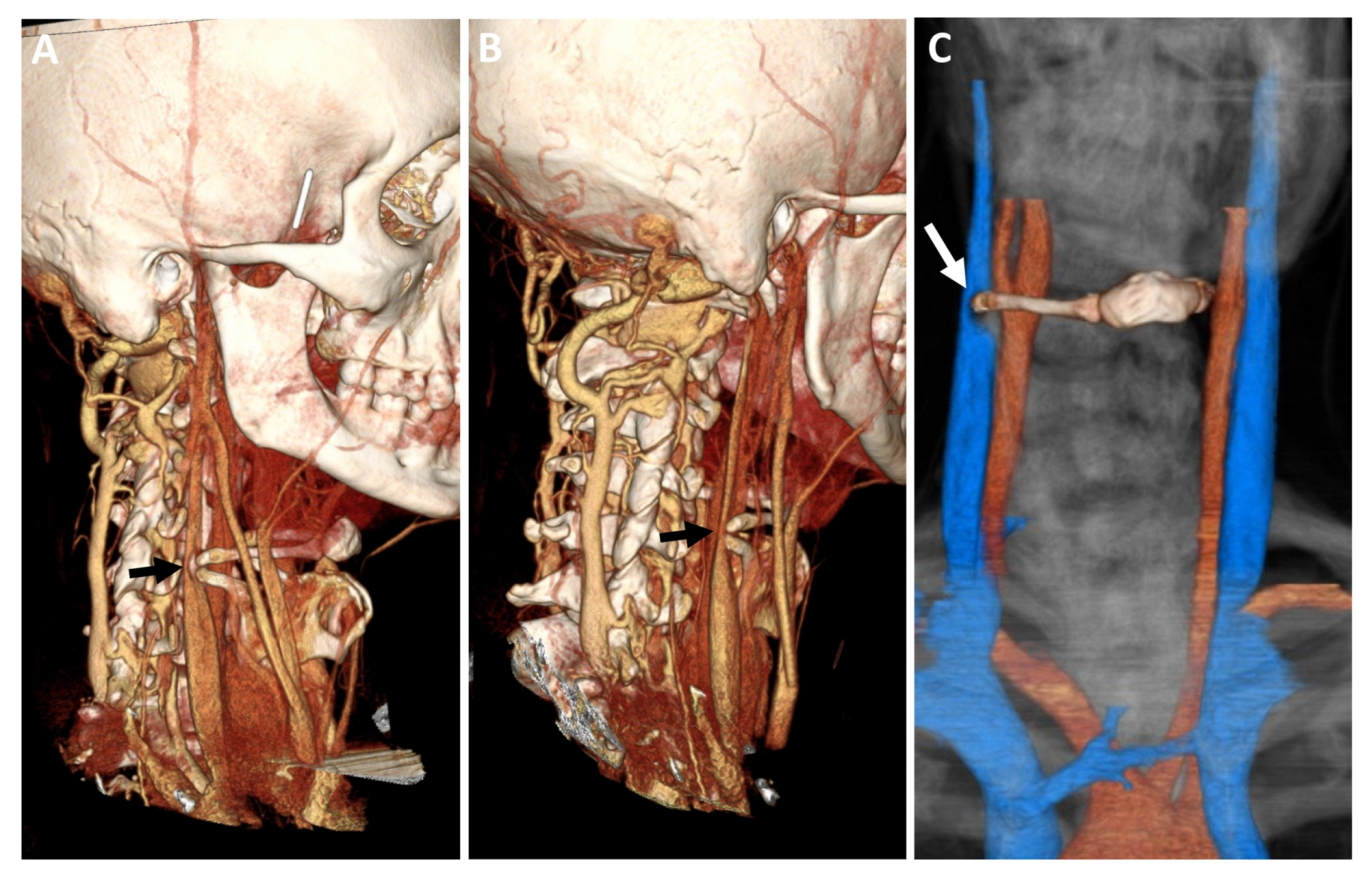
Three-dimensional reconstruction of the preoperative vessel imaging. (A, B, C) Three-dimensional reconstruction of the preoperative CTV clearly demonstrating extrinsic compression of the IJV by a hypertrophied hyoid bone and superior thyroid cartilage (black arrow in A, B; white arrow in C). CTV, computed tomography venogram; IJV, internal jugular vein
